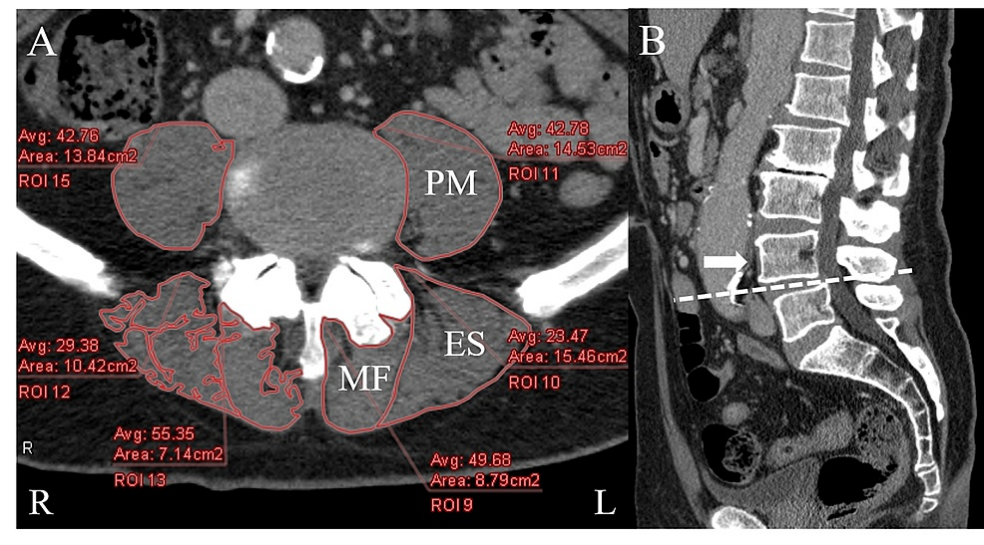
Measurement of the functional cross-sectional area and gross cross-sectional area of the lumbar muscles (A) Axial reconstructed CT images. Measurements of the functional cross-sectional area of the right-side PM, ES, and MF muscles are shown. Measurements of the gross cross-sectional area of the left-side PM, ES, and MF muscles are shown. (B) Sagittal reconstructed CT images showing L4 degenerative spondylolisthesis. The level from which measurements are taken (white arrow) was the disc level at L4-L5 parallel to the inferior endplate of L4 (white dotted line). PM, psoas major; ES, erector spinae; MF, multifidus; L, left; R, right
